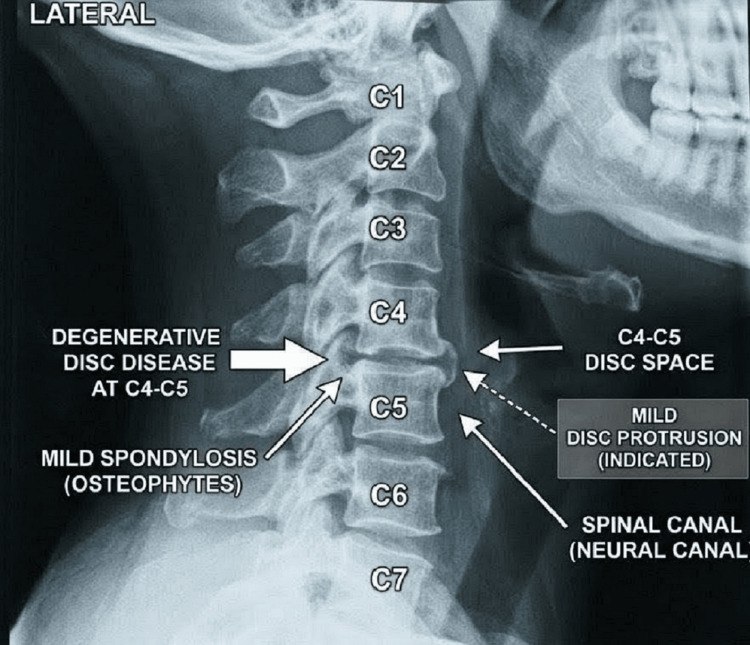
Cervical spine X-ray, lateral view, demonstrated changes at the C4-C5 level with disc protrusion and mild spondylosis.
